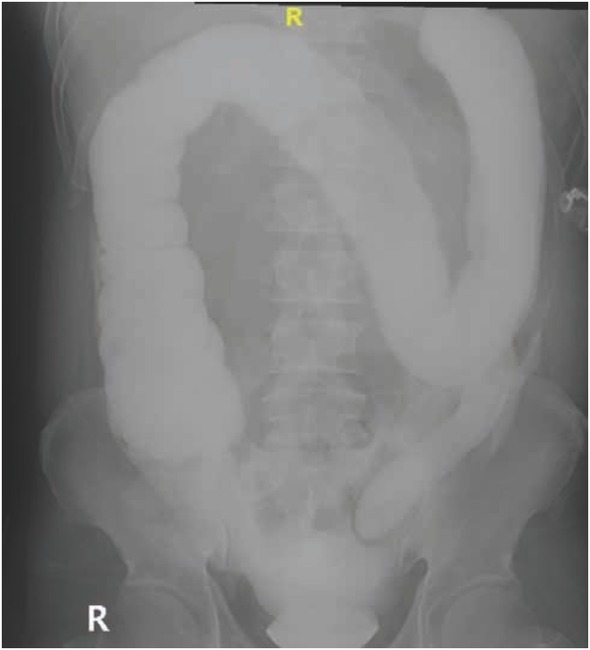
(Case 2) Plain abdominal x-ray done eight hours after the distal loopogram procedure showed extraluminal contrast.
